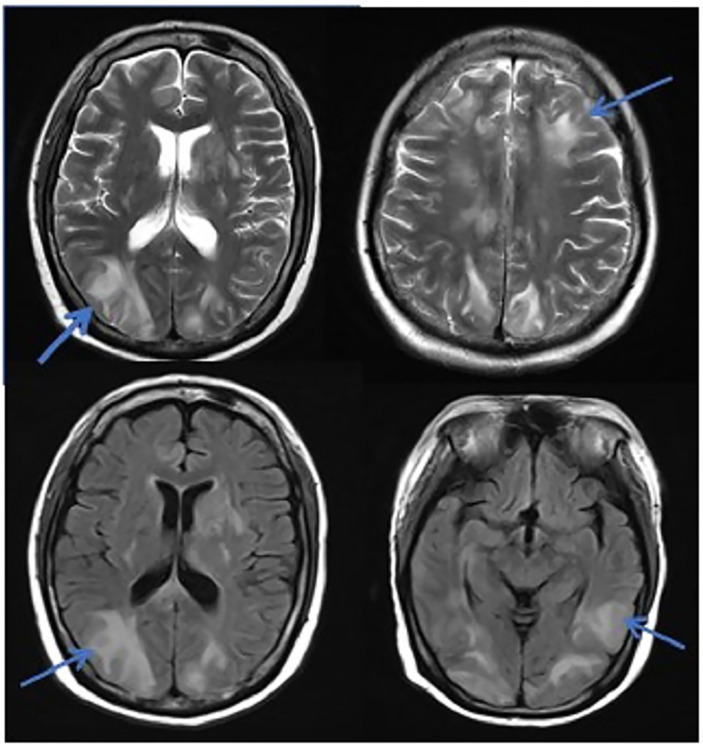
MRI in patients with PRES. The patient diagnosed with PRES showed a patchy low-intensity T1WI on the left occipital lobe, a high-intensity shadow on the T2WI and water pressure image, a high-intensity shadow in the DWI sequence part, unclear borders, and a shallow fission near the sulci.
